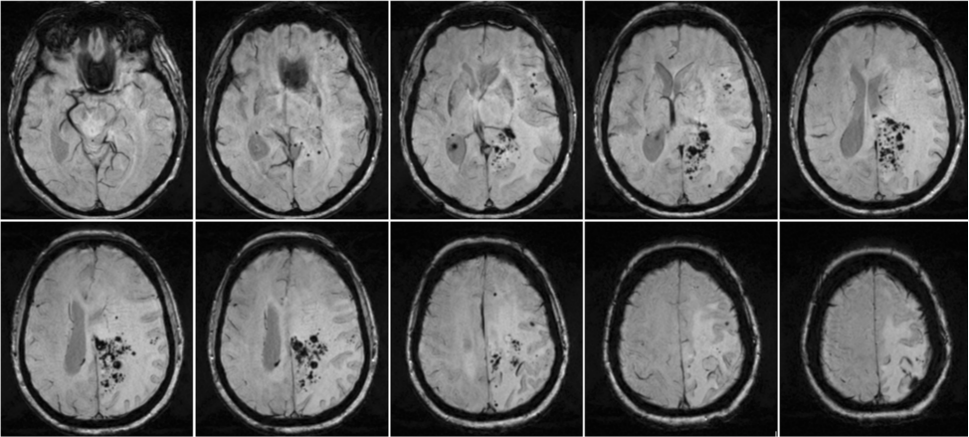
Susceptibility-weighed (SW) sequence of the initial MRI
